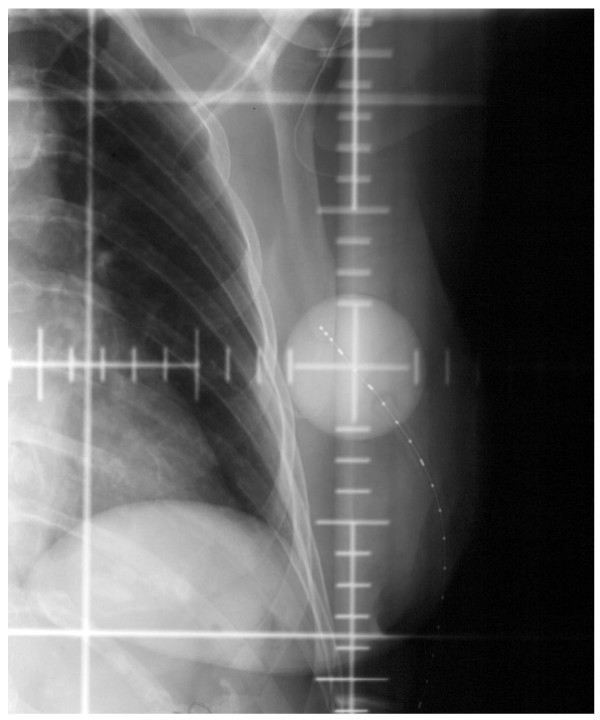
AP radiograph demonstrating the image reviewed for daily quality assurance measurements of the MammoSite balloon diameter. The catheter contains a radio-opaque strand with markers at intervals of 1 cm.
